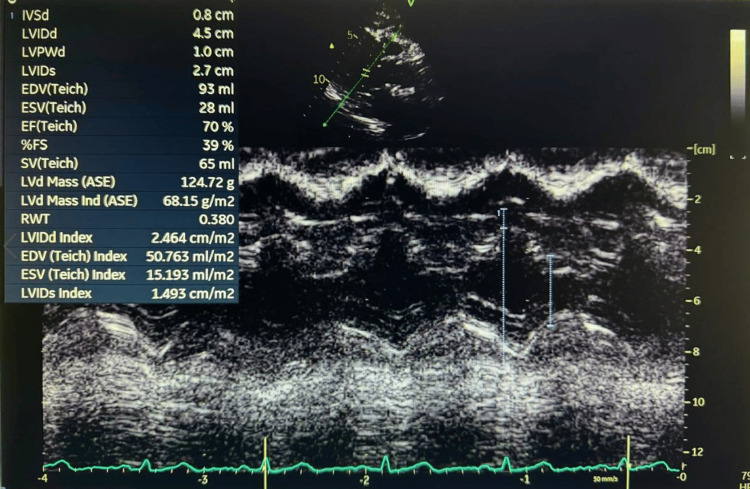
M-mode transthoracic echocardiography demonstrating preserved left ventricular systolic function. The calculated ejection fraction (EF) is 70%, and the fractional shortening (FS) is 39%, indicating normal contractility. Left ventricular dimensions and wall thicknesses are within normal reference ranges. IVSd: interventricular septal thickness in diastole; LVIDd: left ventricular internal diameter in diastole; LVPWd: left ventricular posterior wall thickness in diastole; LVIDs: left ventricular internal diameter in systole; EDV: end-diastolic volume; ESV: end-systolic volume; EF: ejection fraction; FS: fractional shortening; SV: stroke volume; LVd: left ventricular diameter; ASE: American Society of Echocardiography; RWT: relative wall thickness
